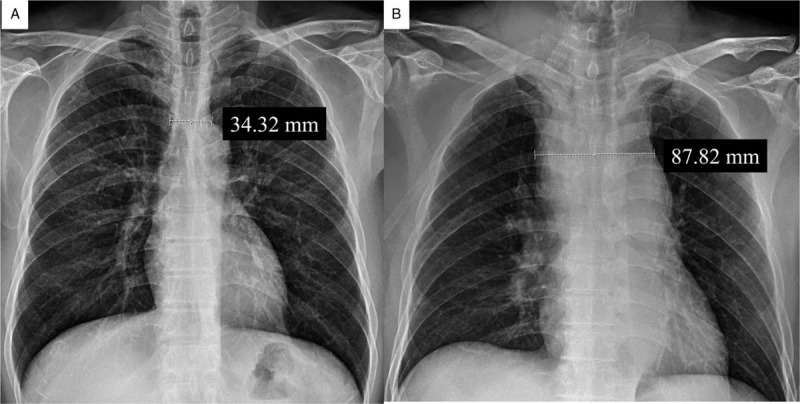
Chest X-ray. A. The pre-interventional image shows the normal range of mediastinum and chest. B. The post-interventional image shows the newly developed mediastinal widening. The numbers indicate the mediastinal size just above the aortic arch level.
